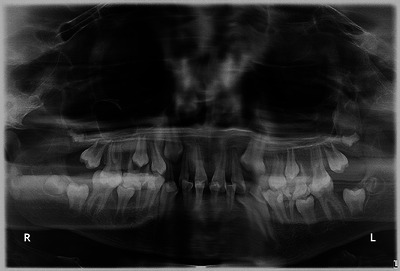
Panoramic radiograph showing mixed dentition. Taurodontism is observed, particularly in teeth 16 and 26, characterized by enlarged pulp chambers and apical displacement of the root furcation. Shortened roots are also evident in the mandibular permanent incisors (teeth 31, 32, 41, and 42). Increased pericoronal space is associated with teeth 13 and 45.
